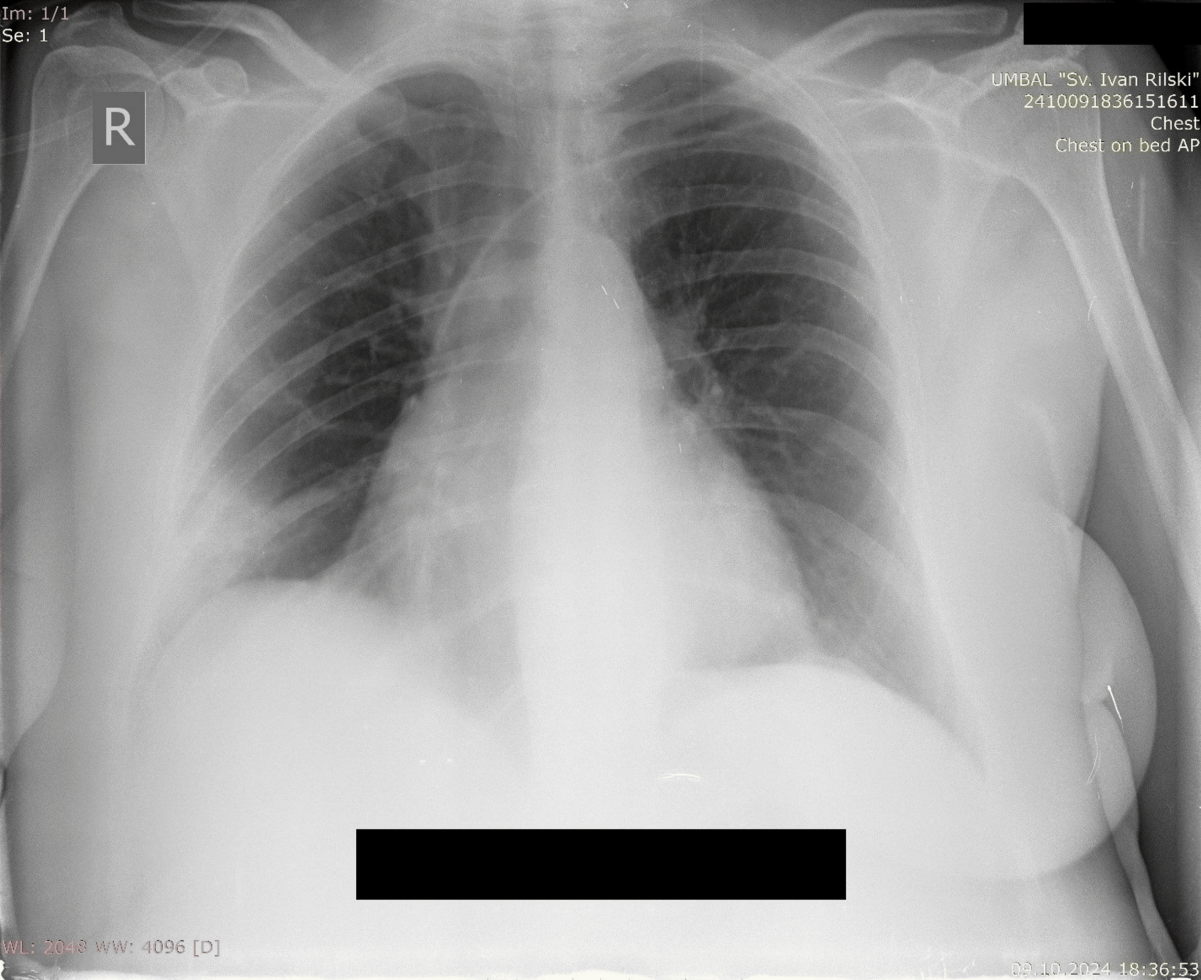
Chest X-ray at admission showing opacification in the right lower lung field
